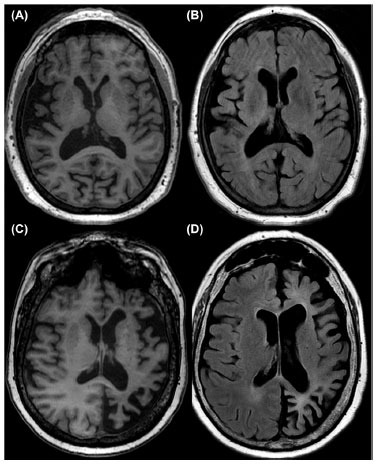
Brain MRI of two sisters with hemiconvulsion-hemiplegia syndrome (IHHS). Note the cortico-subcortical atrophy of the right hemisphere (A, B) in the 64-year-old proband, while the brain atrophy was contralateral and limited to the left hemisphere (C, D) in her 58-year-old sister.
